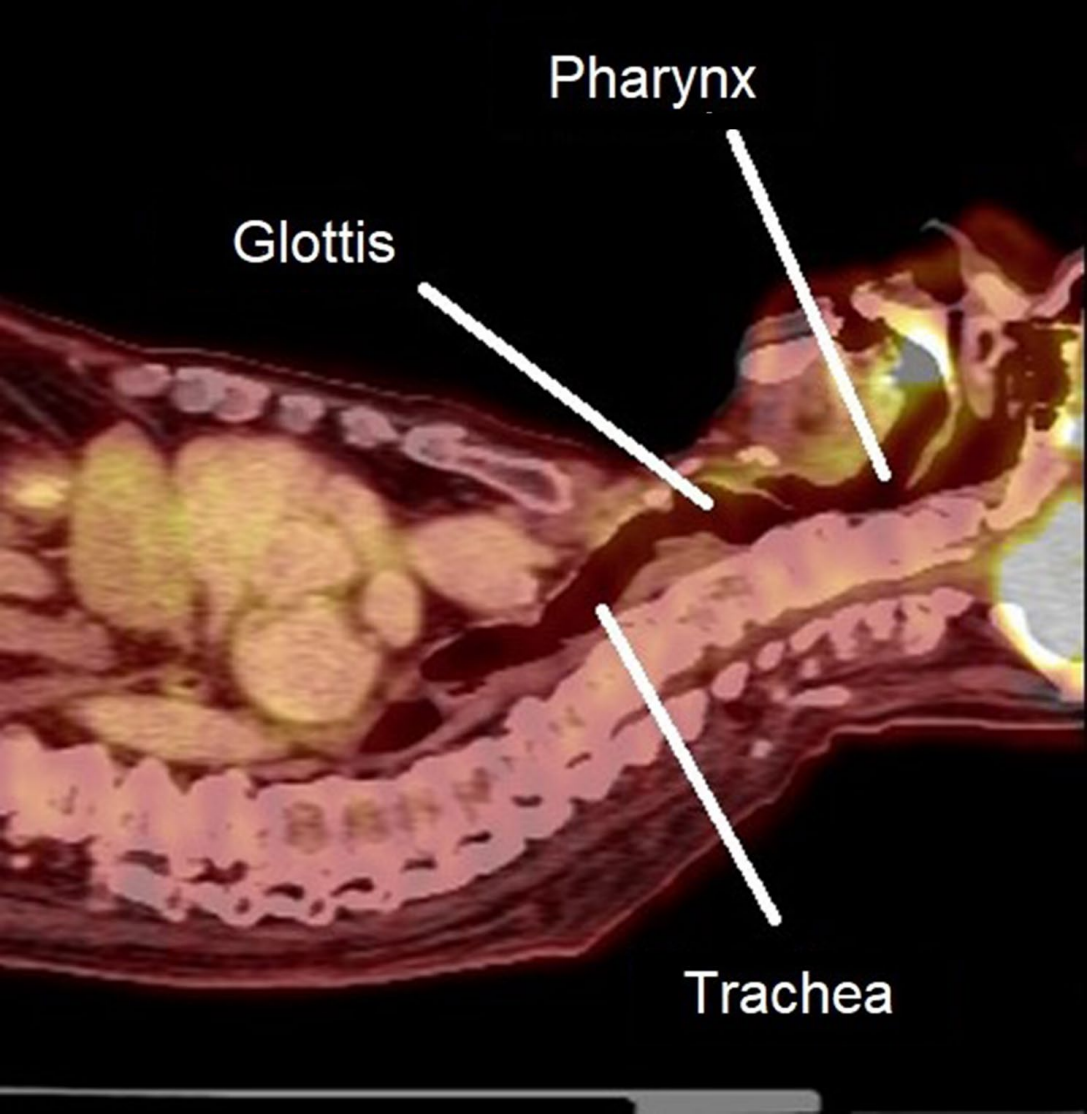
A fused sagittal image from a PET-diagnostic CT scan in a plane useful for evaluation of the airway (pharynx, glottis, and trachea)
